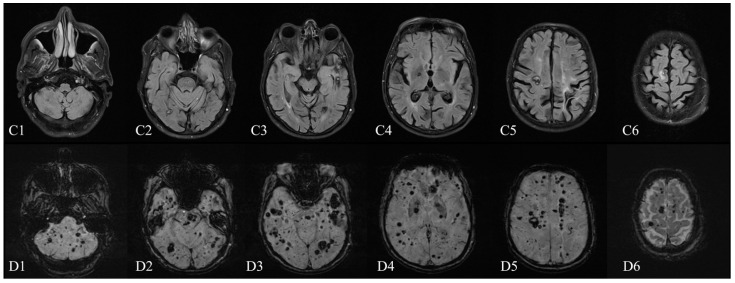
Brain MRI (1.5 T)—January 2021: (C1–C6) axial FLAIR sequence and (D1–D6) axial SWI sequence (TE 40.00 ms). Compared with the 2015 MRI (1.5 T), the number and the dimensions of cavernomas substantially increased in almost every cerebral location (comparing the B1–B6 to the D1–D6 SWI series of imaging). The 2021 cerebral MRI (Figure 2) showed a significant increase in dimensions of many CCMs as well as the addition of novel lesions compared with the previous examination, without signs of acute bleeding. Some of the large CCMs (over 10 mm) identified on the 2015 MRI examination, of the Zabramski I and II subtype, had evolved to the Zabramski III subtype (Figure 3); moreover, countless new Zabramski type IV small lesions were observed [14]. The spinal examination revealed multiple focal lesions at cervical and thoracic levels, also highly suggestive of cavernous malformations (Figure 4C). One of the lesions, at the C3–C4 level, showed signs of recent hemorrhage (Figure 4A,B).
